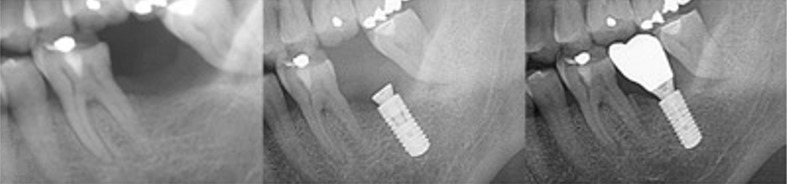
Radiographs taken (a) 40 months after loading, showing statistically significant bone resorption around the implant and (b) 33 months after loading, when the peri-implant bone level was stable.
